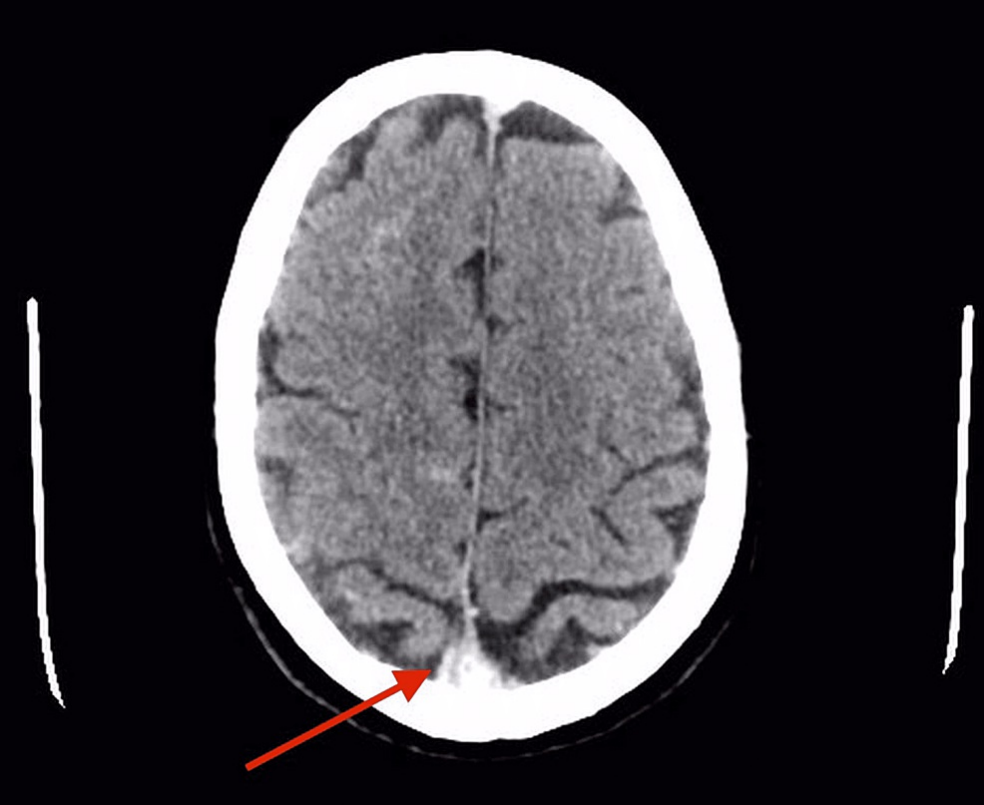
CT plain of the brain (axial section). The red arrow is pointing toward the superior sagittal sinus, which is hyperdense (positive delta sign), indicating there is a thrombus.
